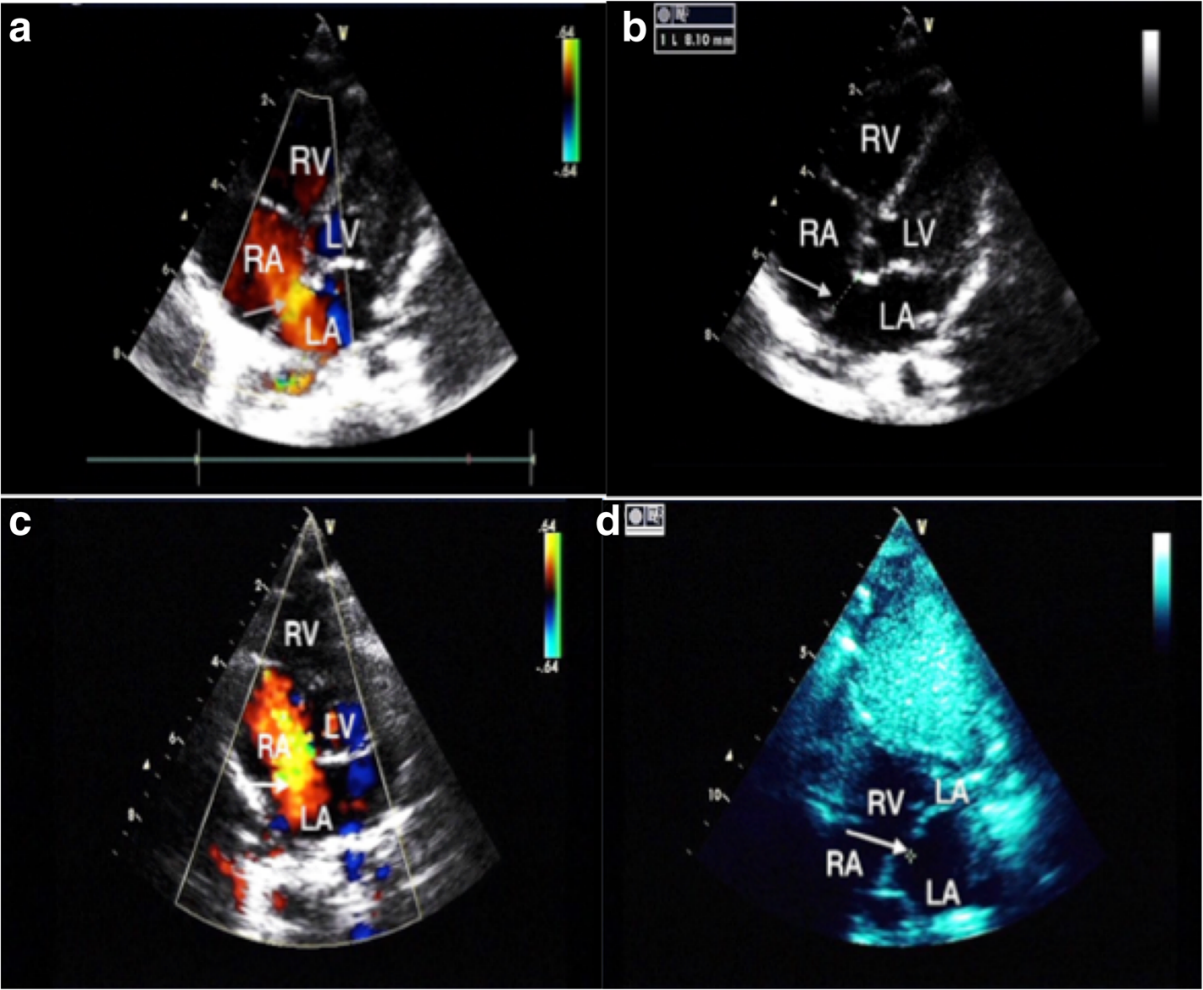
a At our patient’s first visit (8-months old), color Doppler echocardiography showed a secundum defect with left-to-right shunt in the middle of atrial septum and the Qp/Qs ratio was 1.6 which meant the blood shunt was moderate (*arrow*). b On transthoracic echocardiography, we noted that the defect size of atrial septal defect was 8.1 mm (*arrow*). c When he was 3-years old, the left-to-right shunt still existed and the Qp/Qs ratio was 1.9 which meant the blood shunt was moderate to severe (*arrow*). d On transthoracic echocardiography, we noted that the existing defect had increased and the size was 10 mm (*arrow*), the blood shunt and defect size were all increased, which suggested exacerbation of atrial septal defect. *LA* left atrium, *LV* left ventricle, *RA* right atrium, *RV* right ventricle
